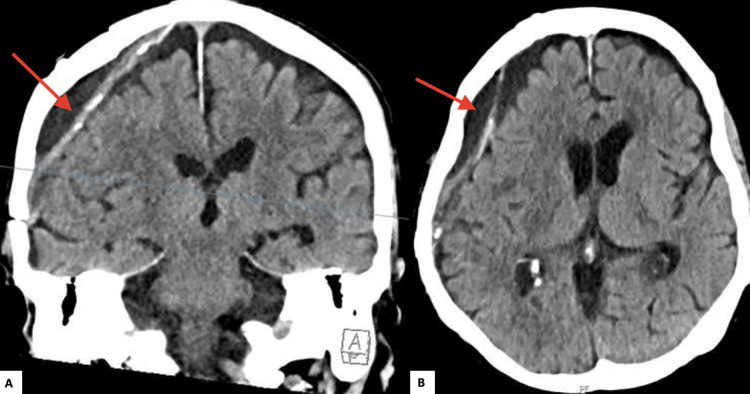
CT scan showing subacute subdural hematoma on the right hemisphere (A: coronal; B: axial). The blue line in the coronal section represents the axial cut section accordingly.
